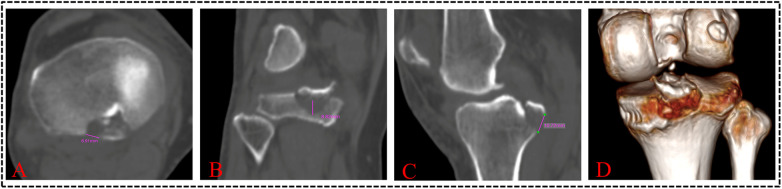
CT and 3DCT reconstruction images of PCL tibial avulsion fracture. (A–C) Axial, coronal, and sagittal CT planes, (D) 3DCT reconstructed images.
